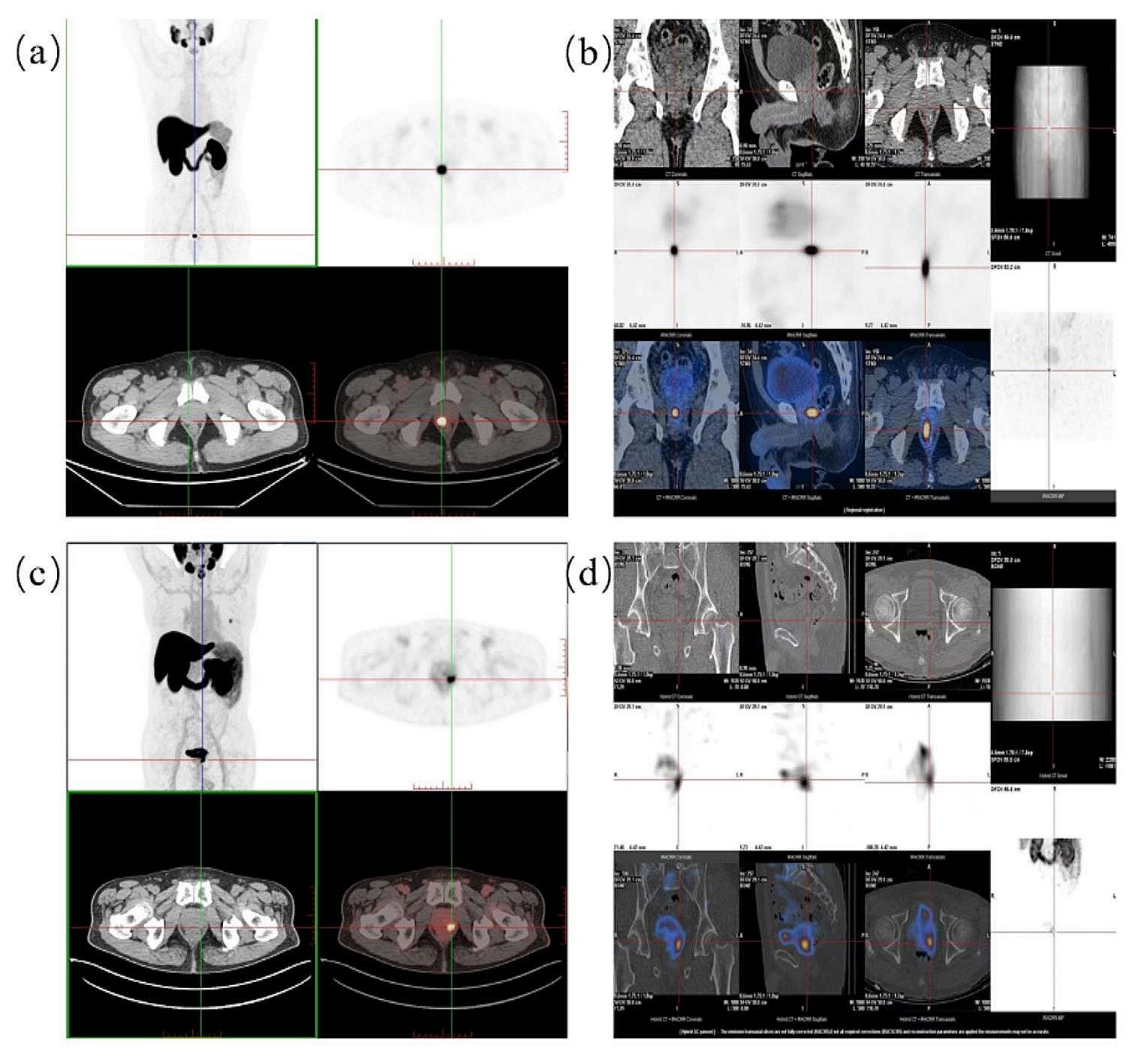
(a) Imaging of [^18^F]F-PSMA-7Q in Patient 11. (b) Imaging of [^123^I]I-PSMA-7 in Patient 11 at 4 h after injection. (c) Imaging of [^18^F]F-PSMA-7Q in Patient 12. (d) Imaging of [^123^I]I-PSMA-7 in Patient 12 at 4 h after injection
